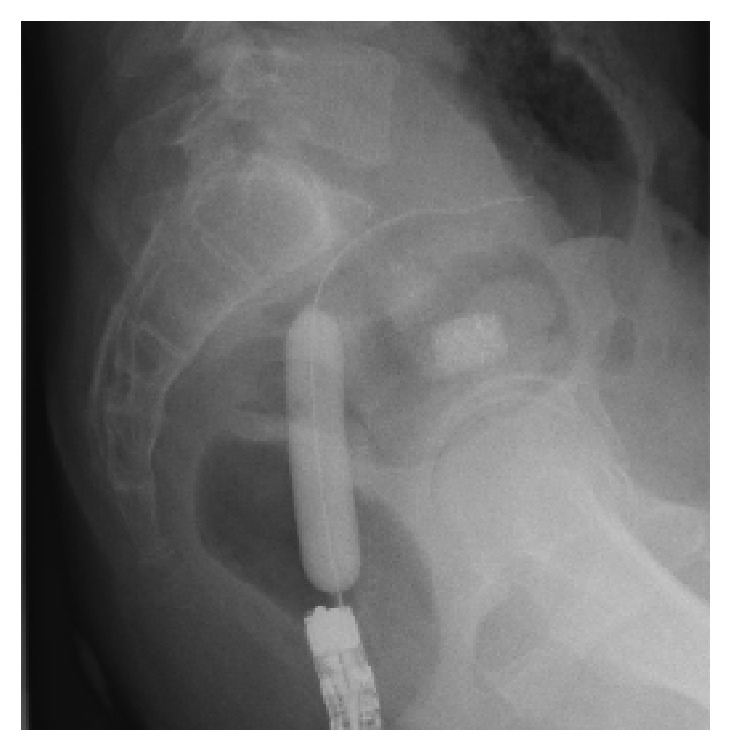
Abdominal radiograph depicting the endoscopic balloon dilatation for the stenotic lesion between the rectum and the sigmoid colon.
